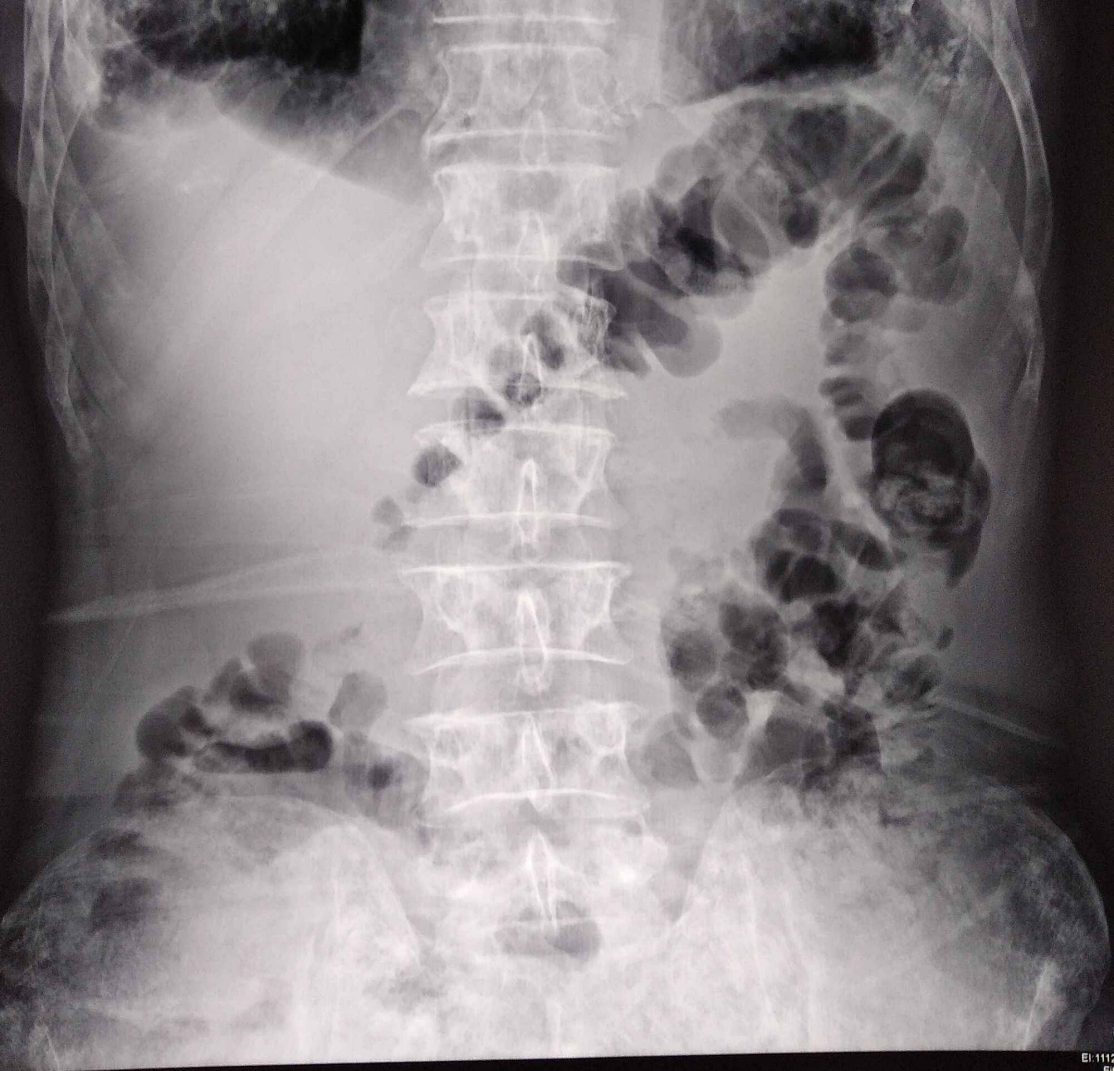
No significant finding on abdominal X-ray
